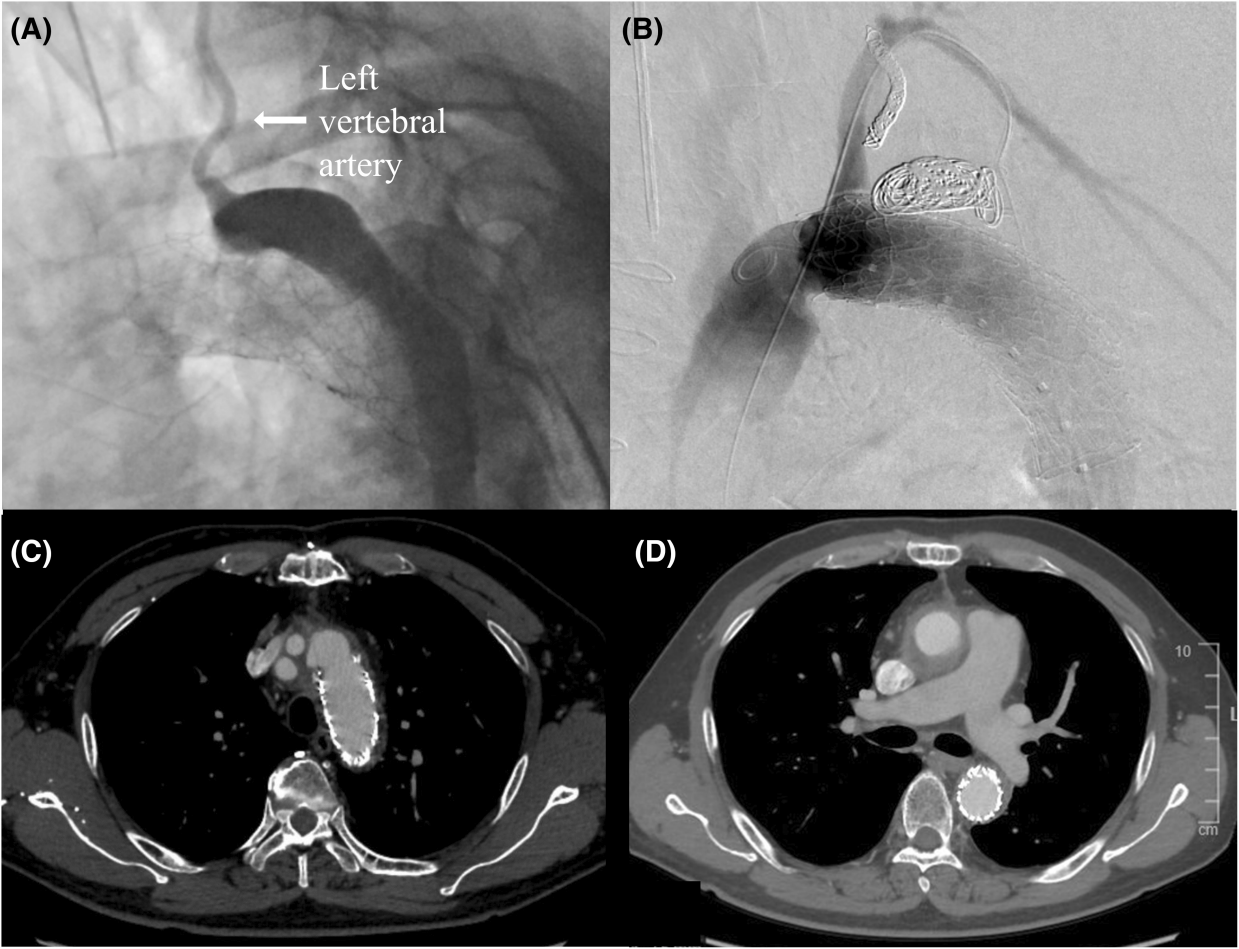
(A) Intraoperative angiography showing a patent left vertebral artery. (B) Post‐treatment angiography reveals that the antegrade blood flow into the left subclavian artery from the fenestration in the frozen elephant trunk is preserved and no endoleaks. (C,D) A 1 year post‐treatment computed tomography shows the false lumen had shrunken
